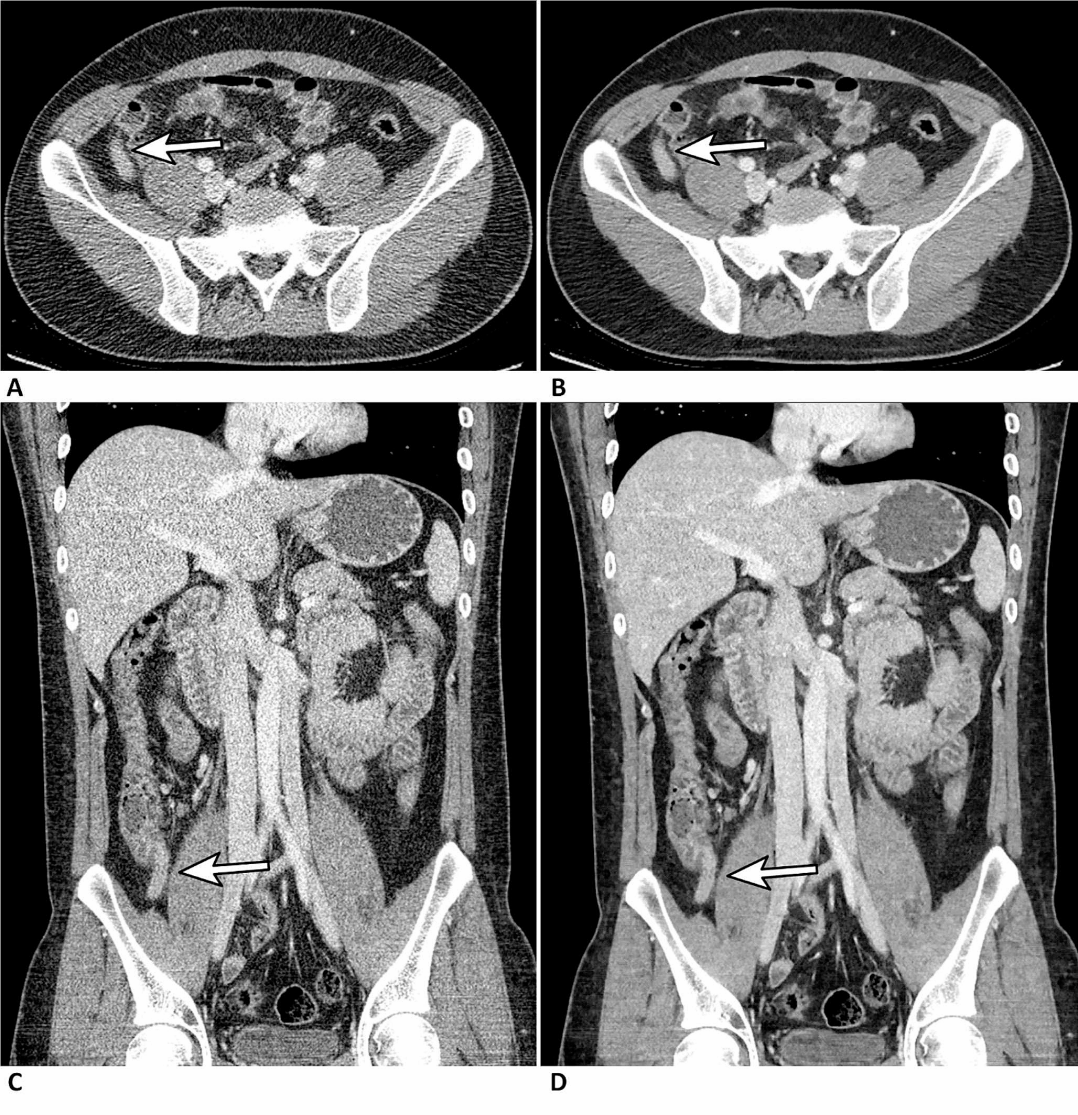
A 29-year-old man underwent CT examination for suspected appendicitis (64-channel CT from GE). Ultralow-dose CT images (a, c) and ultralow-dose CT with deep-learning denoising algorithm (b, d). The patient was confirmed with appendicitis through an appendectomy
